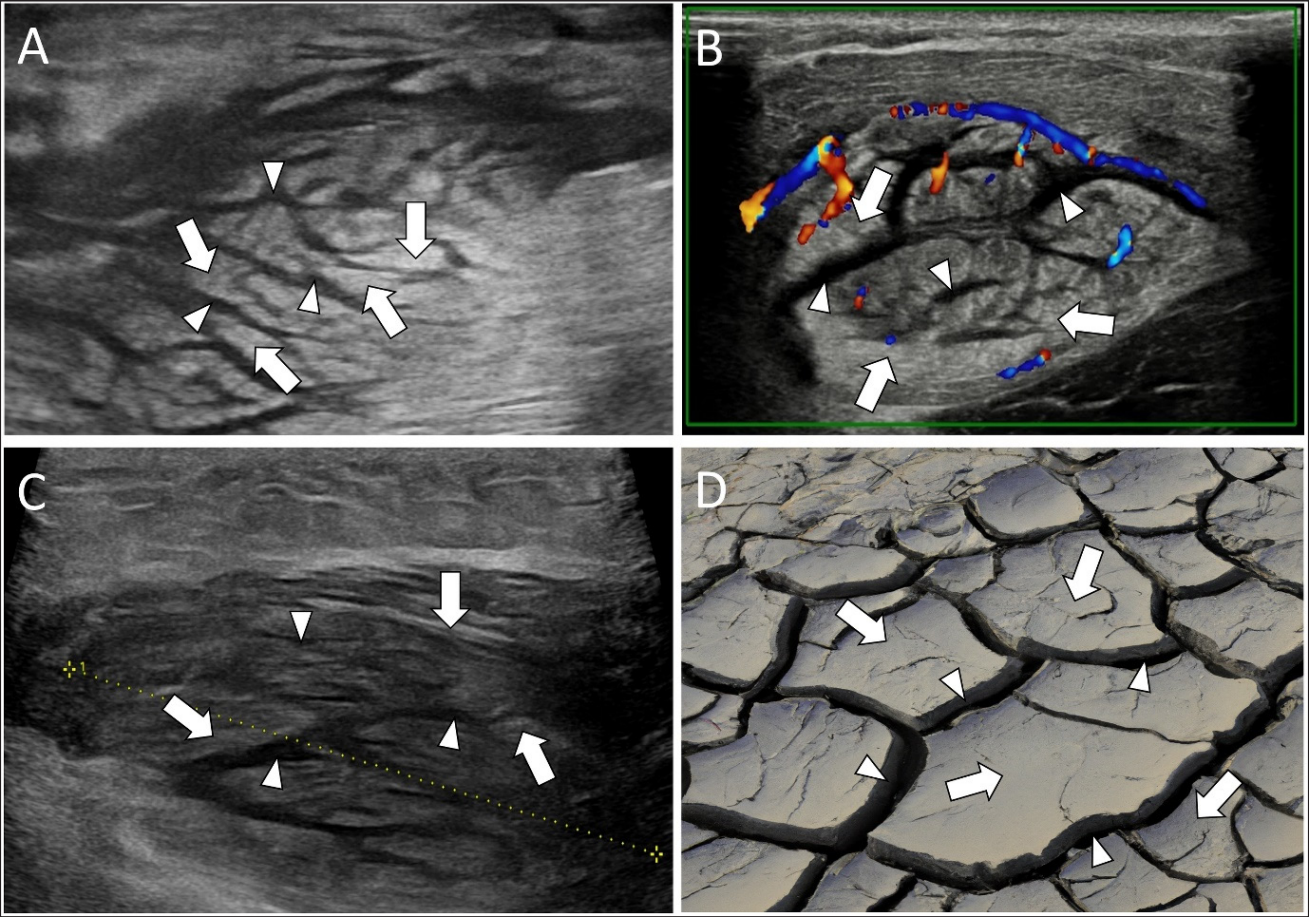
US showing a ‘cracked dry mud’ pattern of PM with swollen hyperechoic muscle fibers (white arrows) interspersed with hypoechoic bands (white arrowheads). A: Transverse image of the right brachialis muscle of patient 1. B: Transverse color Doppler image of the left brachioradialis muscle of patient 2 showing hypervascularity. C: Longitudinal image of the right deltoid muscle of patient 3. D: ‘Cracked dry mud’.
